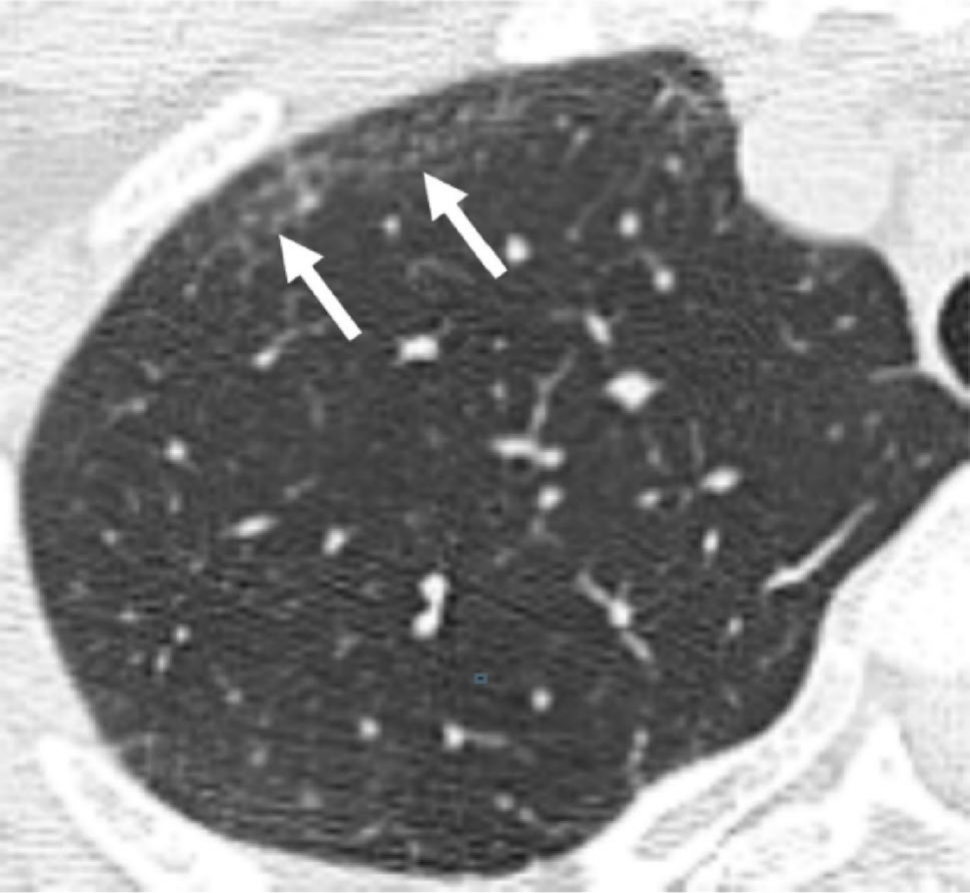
A 39-year-old woman with FANO. Unenhanced axial chest HRCT shows small, faint nodules on amorphous ground-glass opacity within 1 cm of the pleura, with a band-like distribution parallel to the pleura. The finding is particularly prominent along the anterolateral portions (arrows). *FANO* faint amorphous nodular opacity, *HRCT* high-resolution CT
